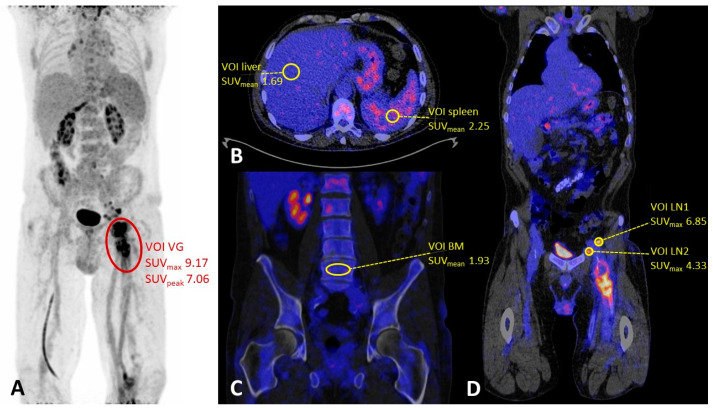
(A) Maximum intensity projection (MIP) of a patient with a proven femoral vascular graft infection (grade 3, heterogeneous uptake) with multiple lymph nodes on the suspected external and common iliac lymphatic drainage pathway. (B) SUV_mean_ measurements in the liver and the spleen. (C) Bone marrow SUV_mean_ measurement in the lumbar spine (L5). (D) SUV_max_ measurements in the lymph nodes. Abbreviations: VOI = volume of interest, VG = vascular graft, BM = bone marrow, LN = lymph node.
